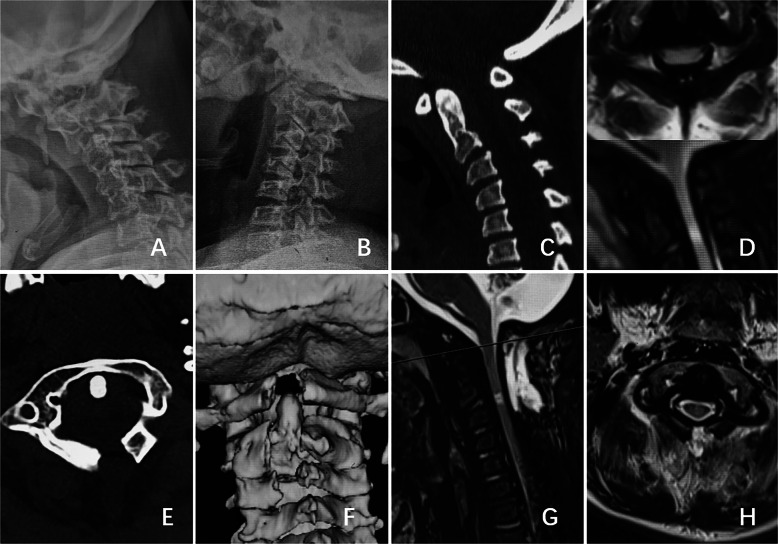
Imaging findings of a 17-year-old patient diagnosed with Type IVA MPS for more than 10 years was admitted to the hospital due to progressive decline in exercise endurance and significant restricted fine motor skills. A, B, C Preoperative dynamic radiograph and CT showed no significant atlantoaxial dislocation. D: Preoperative MRI indicating cervical spinal stenosis and spinal cord compression. E, F Postoperative CT showing that the posterior arch of the atlas was successfully resected. G, H Postoperative MRI showing that cervical spinal canal was decompress and the spinal cord compression was relieved. At the most recent follow-up, the patient reported significant improvement in symptoms, with no additional discomfort or new symptoms
